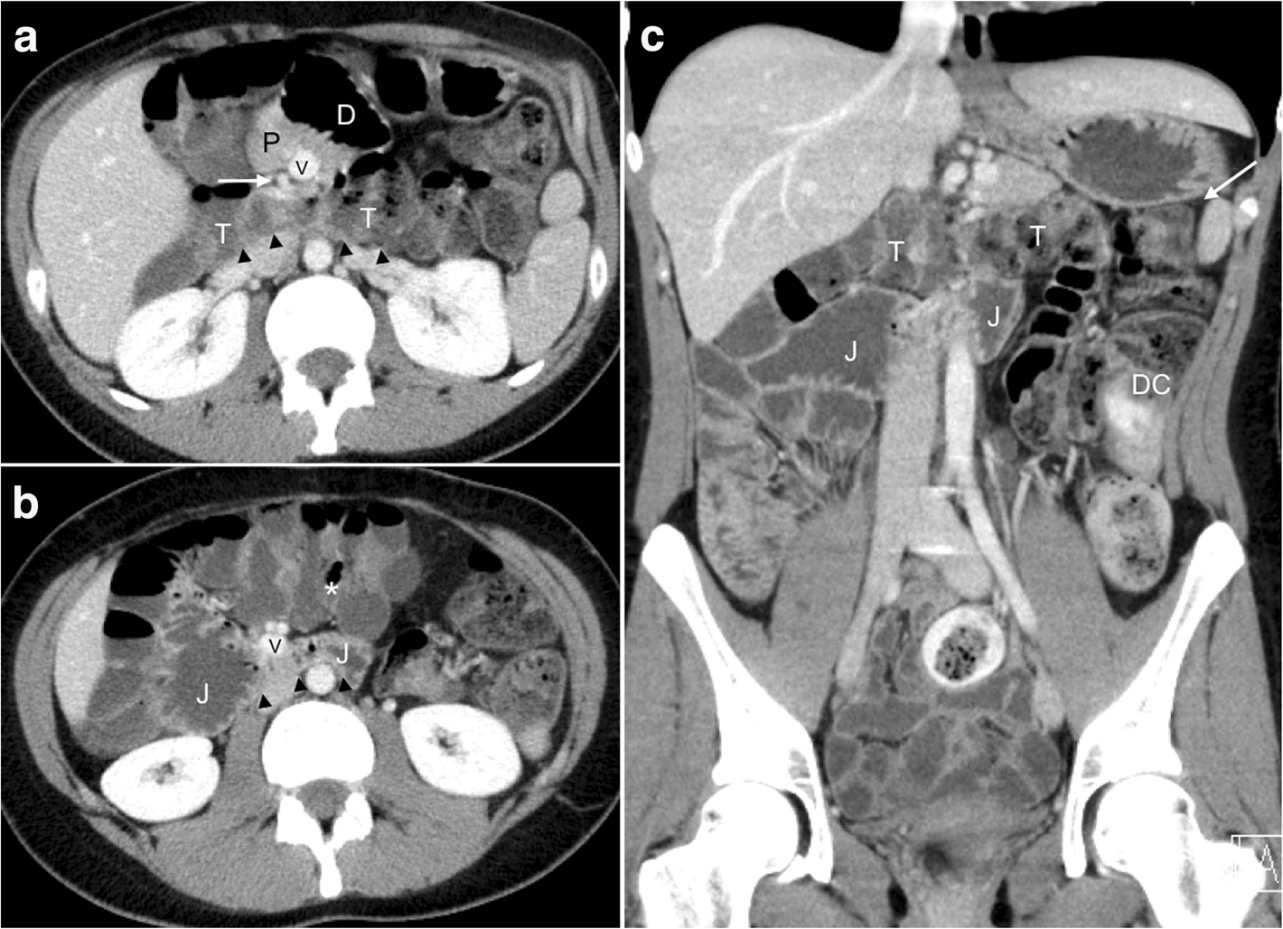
Contrast-enhanced multidetector computed tomography study. a At the level of the pancreatic head (*P*): retro-arterial course (*arrowheads*) of the transverse colon (*T*) dorsal to the mesenteric vessels. Note the inverted relationship of the superior mesenteric vein (*V*) and superior mesenteric artery (*white arrow*) and the aplasia of the pancreatic uncinate process. The duodenum (*D*) crosses from right to left anterior to the pancreas and the mesenteric vessels. b At the level of the posterior-inferior margin of the right hemiliver: retro-arterial course (*arrowheads*) of the proximal jejunum (*J*) from left to right, sandwiched between the superior mesenteric vessels and the aorta. Note the aberrant position of the cecum and the ileocecal valve (*) close to the midline. c Coronal reformatted image: normal position of the left colic flexure (*white arrow*) and the descending colon (*DC*). The relationship between the transverse colon (*T*) and the jejunum (*J*), both in a retro-arterial position, is demonstrated. Several small bowel loops are noted in the lower abdomen
